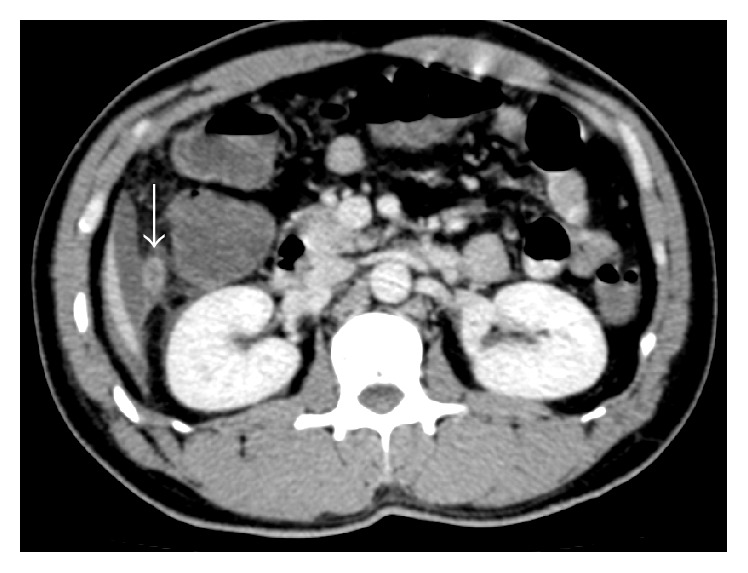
Transverse image of the contrasted CT scan showing the subhepatic appendix (white solid arrow).
